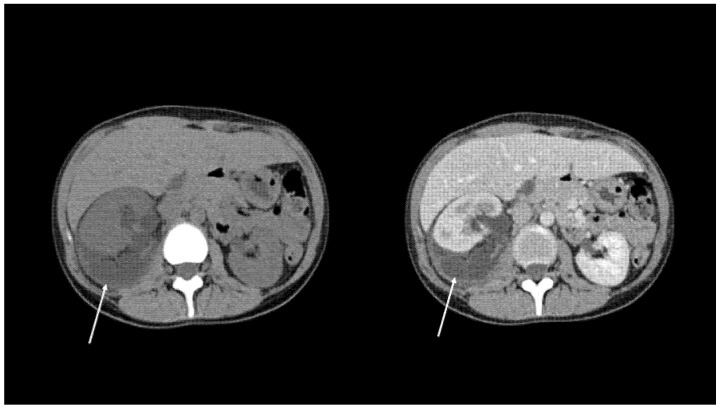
Axial computerised tomography characteristics of urinoma at non contrast CT and delayed excretory phase. Arrows indicate encapsulated fluid collection surrounded by a fibrous capsule.
